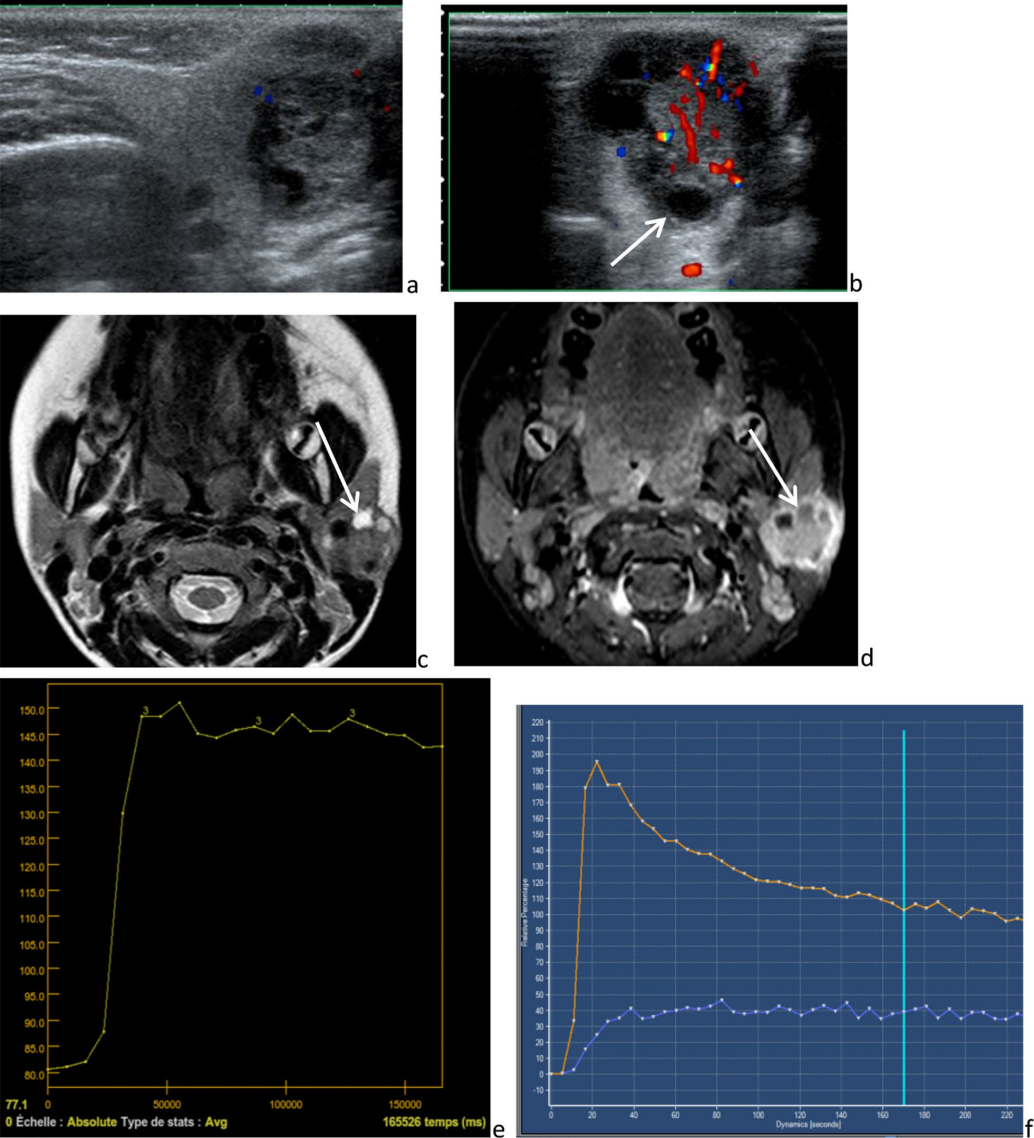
Intermediate-grade mucoepidermoid carcinoma: poorly delineated, heterogeneous tumor containing small necrotic areas [white arrow] and strongly enhanced, on ultrasonography (a and b), axial *T*_2_-weighted image (c) and *T*_1_-weighted image, after intravenous injection of gadolinium chelate (d). Time intensity curve with a signal growing rapidly in the first phase and a low wash out (< 30%): type C or plateau pattern (according to Yabuuchi), suggestive of malignancy (e). Conversely, time intensity curve of type B (orange line), with a high wash out (> 30%), in a case of parotid benign lymphadenopathy (f)
